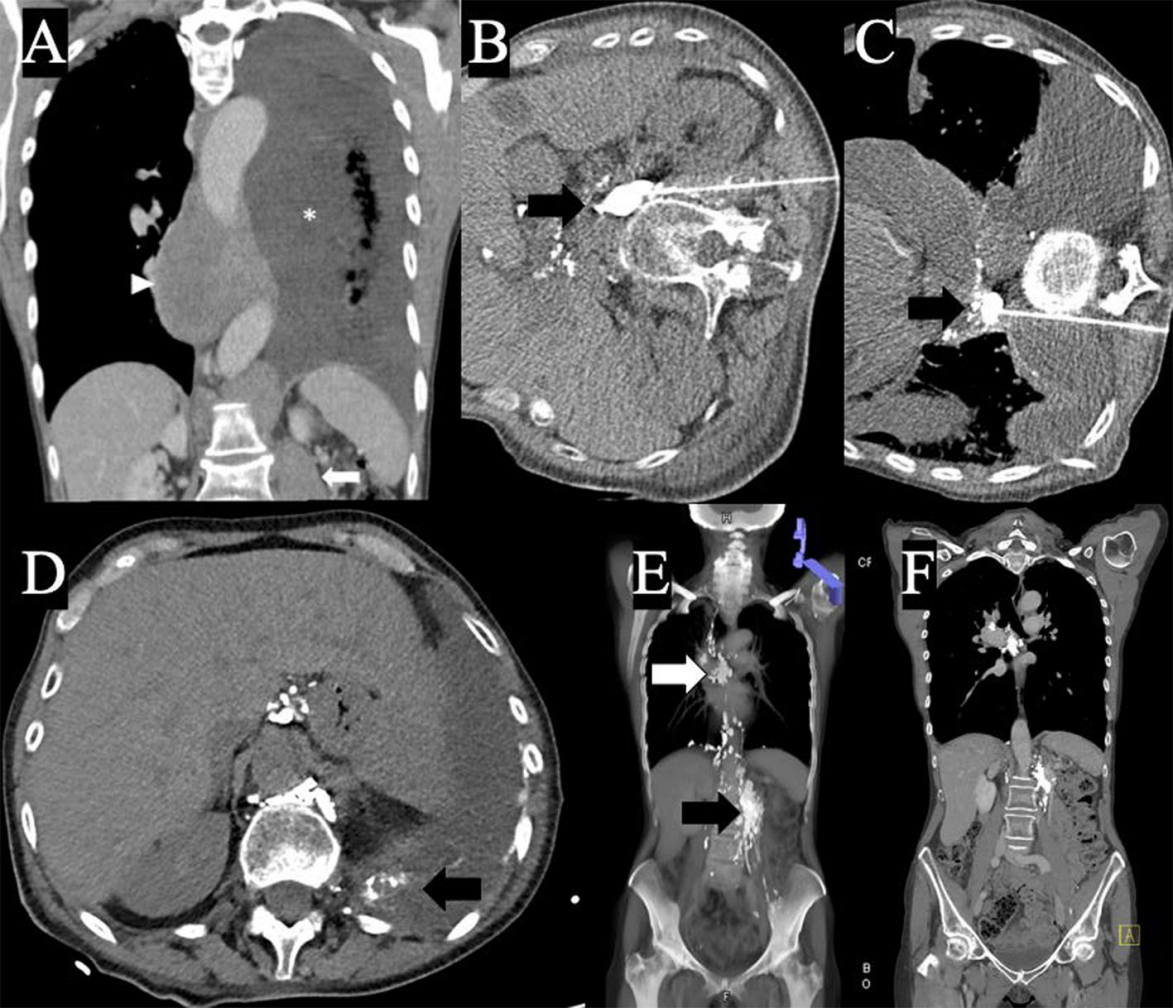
A Pretreatment coronal contrast-enhanced CT image shows thoracic (arrowhead) and abdominal (arrow) large lymphoma bulks and pleural effusion (asterisk). CT-fluoroscopic image shows canulation of B abdominal and C mediastinal lymphoma bulk (arrow) percutaneously with a needle and collection of injected lipiodol in bulk D control scan at the end of the procedure showed lipiodol-glue accumulation within bilateral pleural effusion (arrow, right side not shown) E coronal maximum intensity projection image two weeks after the procedure shows no pleural effusion. Note the residual lipiodol depositions within the shrunken lymphoma bulks (arrows) and lymph vessels in which lipiodol transported antegrade F 1-year follow-up CT image shows complete remission of the lymphoma with no pleural effusion while still showing residual lipiodol deposition
